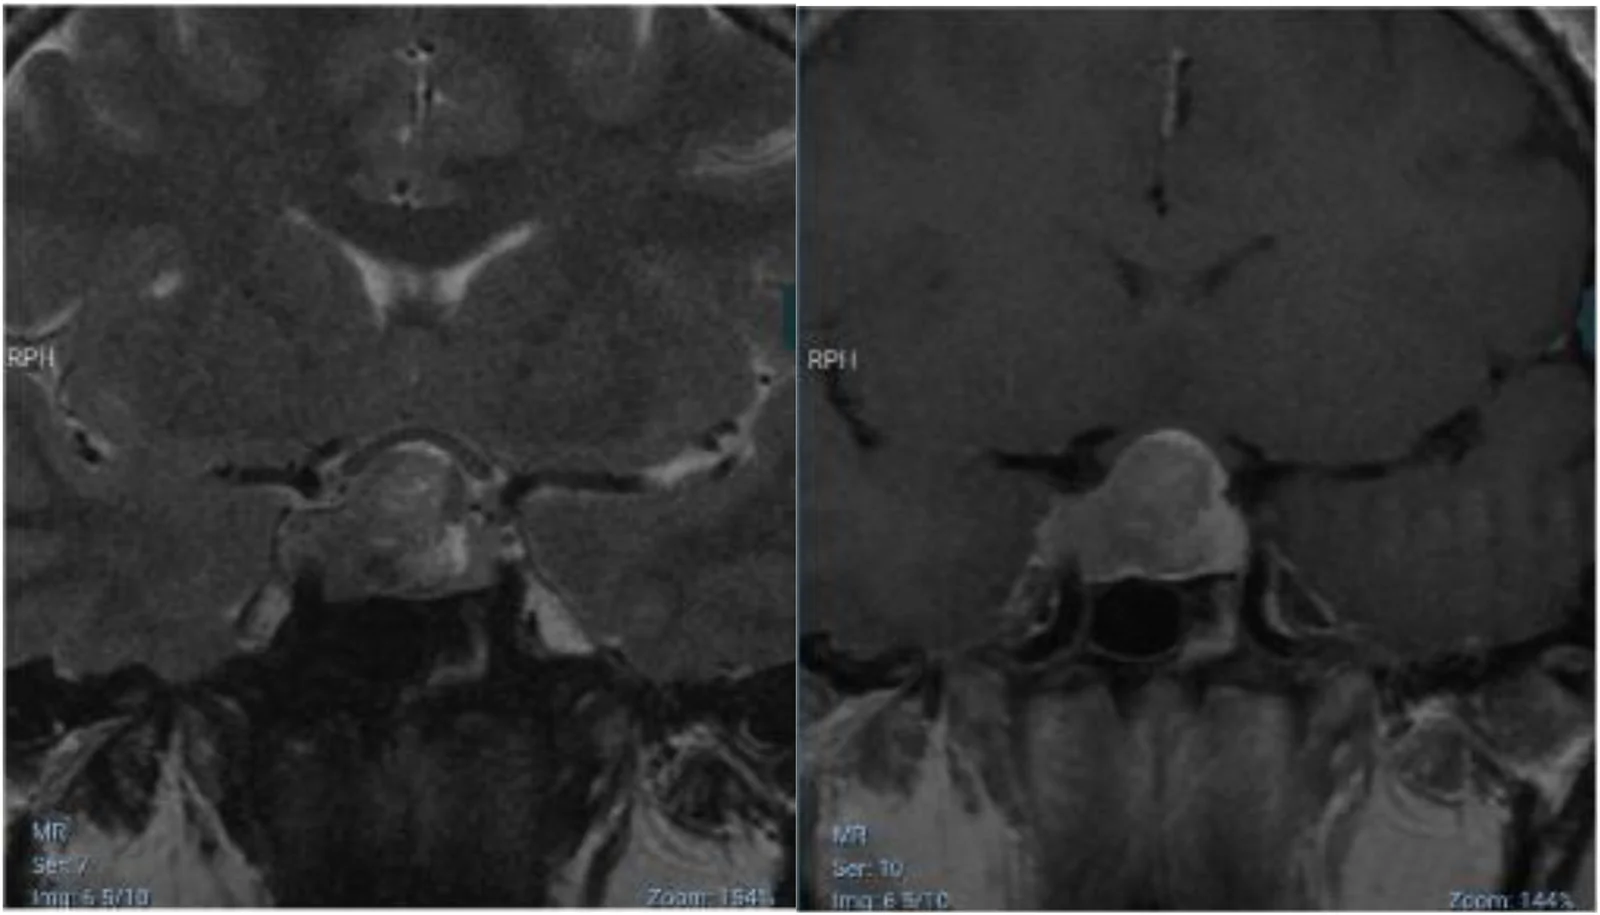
Magnetic resonance imaging of patient 1: pituitary adenoma showing a heterogeneous hyperintense signal in the T2-weighted sequence with invasion to the right cavernous sinus.
